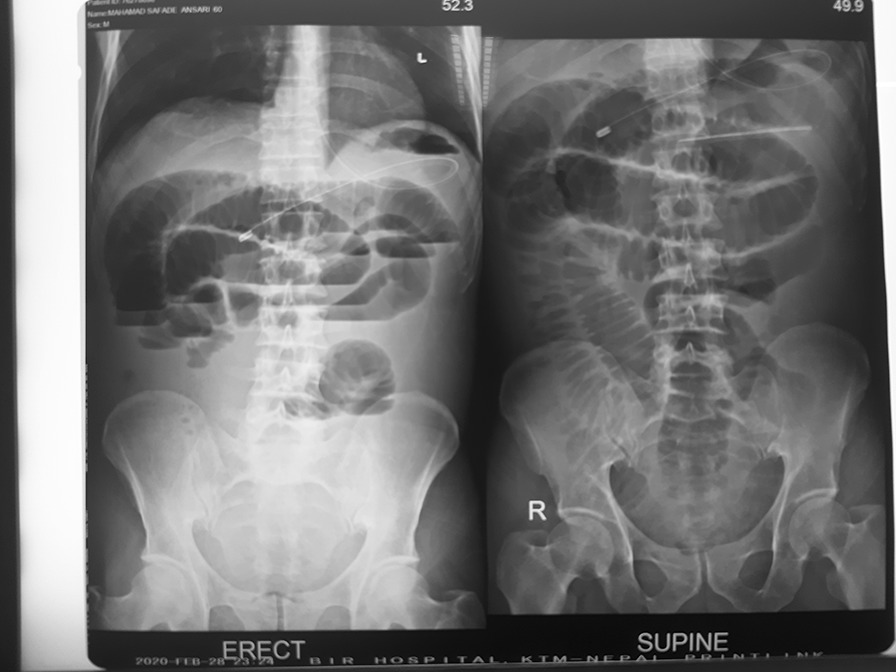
Plain X-ray abdomen showing dilated bowel loops, multiple air-fluid levels and absence of gas in colon
